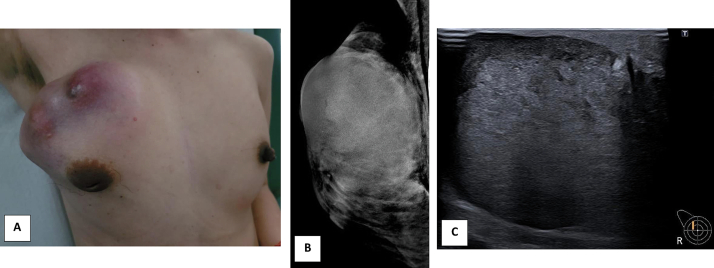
Clinical and imaging techniques. A. Mass with a bluish spot on the skin in the upper quadrant of the right breast. B. Mammogram C. Ultrasound of the lesion in the right breast.
